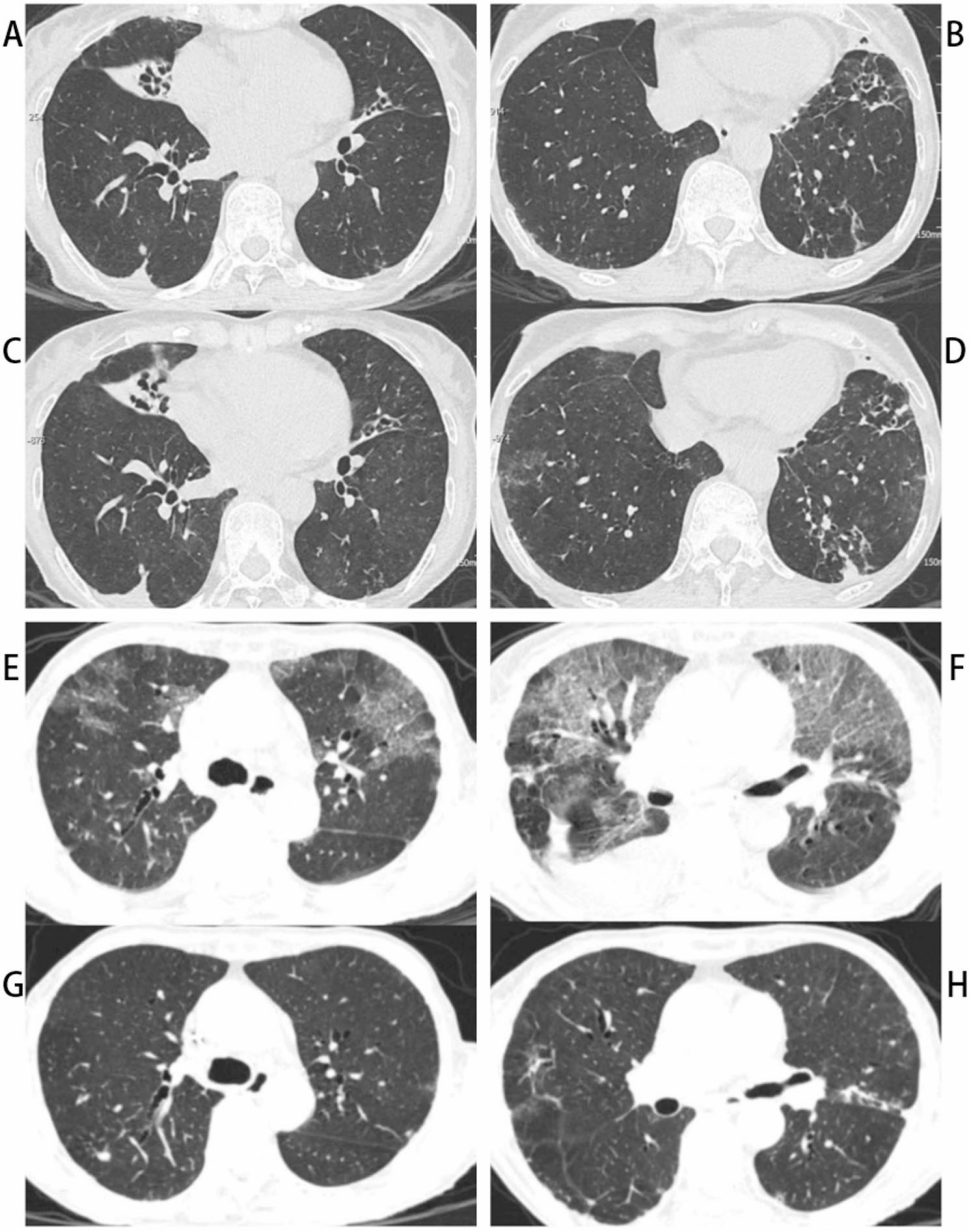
Chest CT scans of the patient. (A, B) Pulmonary window of lung CT on June 17, 2024. (C, D) Lung CT scan on August 15 showed new patchy opacities emerged in the left lower lobe. (E, F) Lung CT scan showed diffuse bilateral ground-glass opacities with interlobular and intralobular septal thickening, accompanied by bilateral pleural effusions on August 26. (G, H) CT scan revealed the regression of the changes in the lungs after treatments on September 6, 2024
